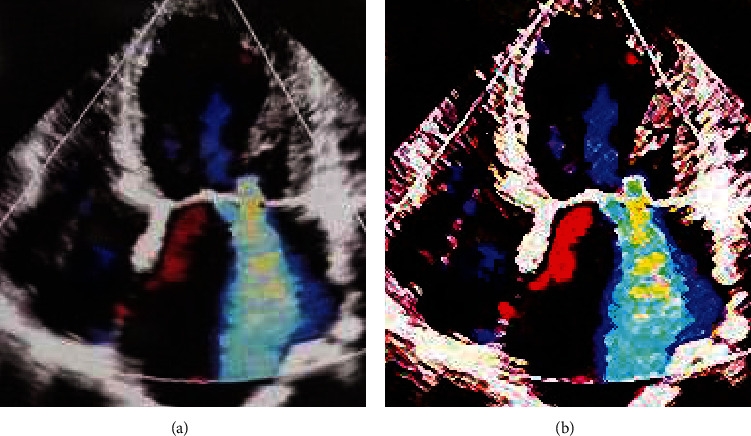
Comparison on cardiac color ultrasound images between the two groups of patients with other heart diseases. (a) Control group; (b) experimental group.
